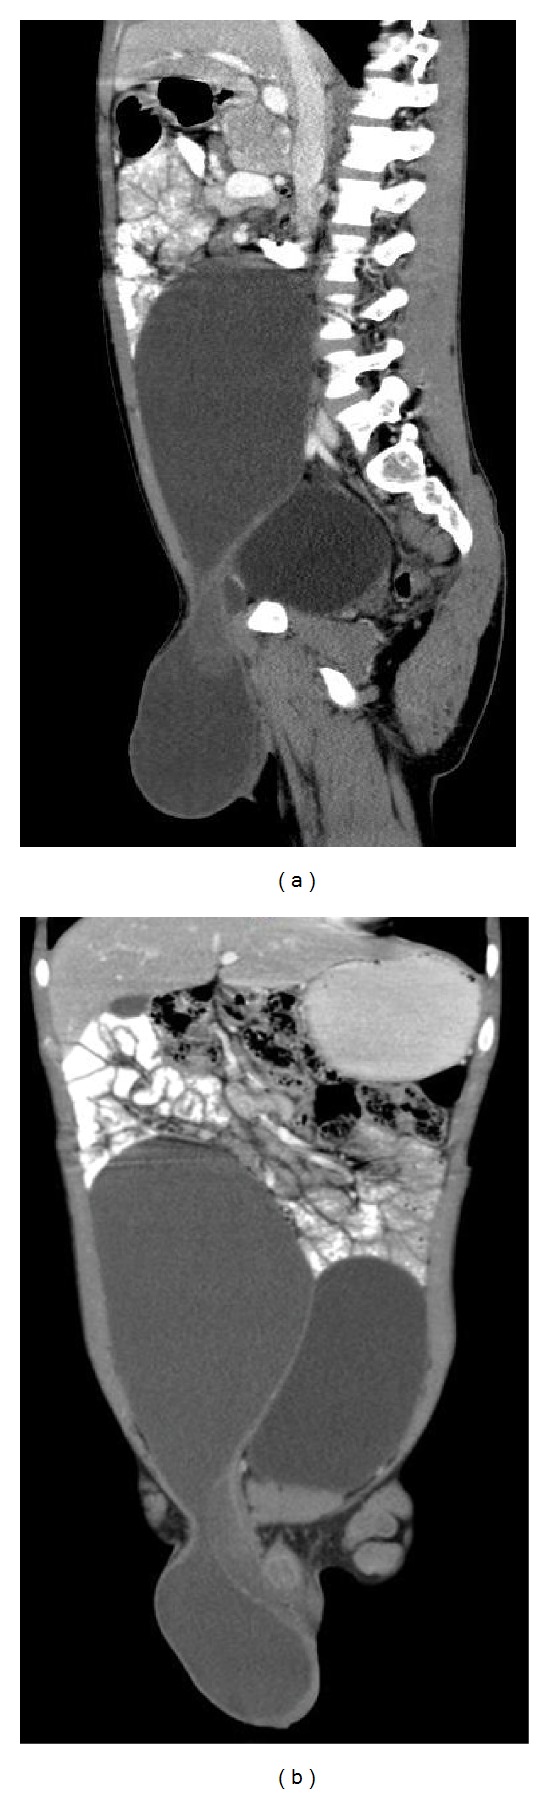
(a) and (b) Coronal and sagittal postcontrast CT images showing a large cystic lesion of thin fluid attenuation in right iliac fossa and lumbar region extending into right scrotal sac. Right testis is located in the right inguinal canal.
